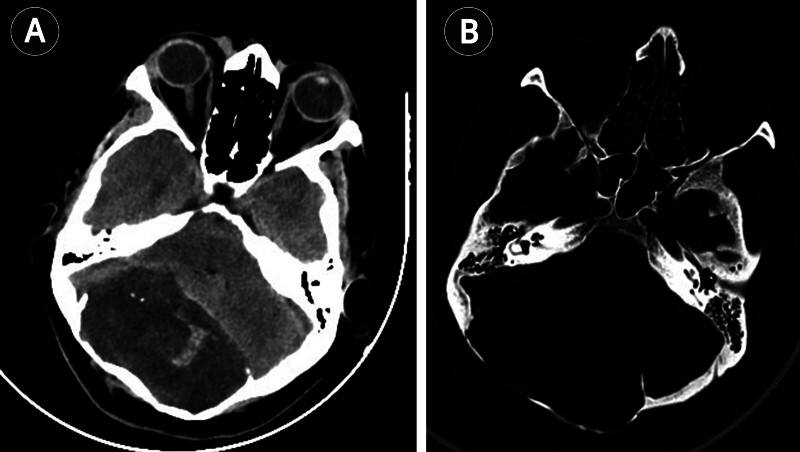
Axial non-contrast CT. (
